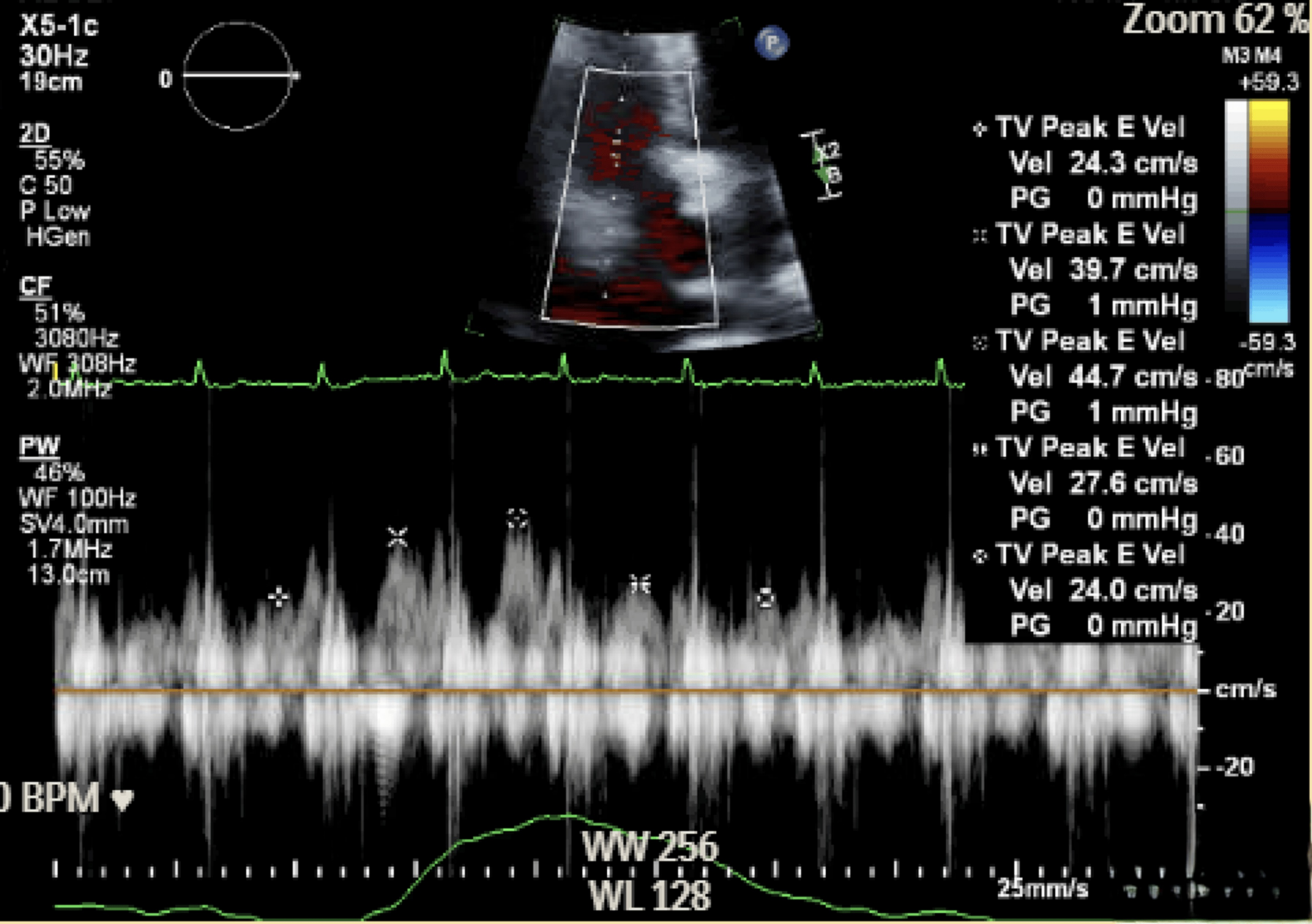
Doppler echocardiography showing marked tricuspid inflow respiratory variation supporting tamponade physiology.
